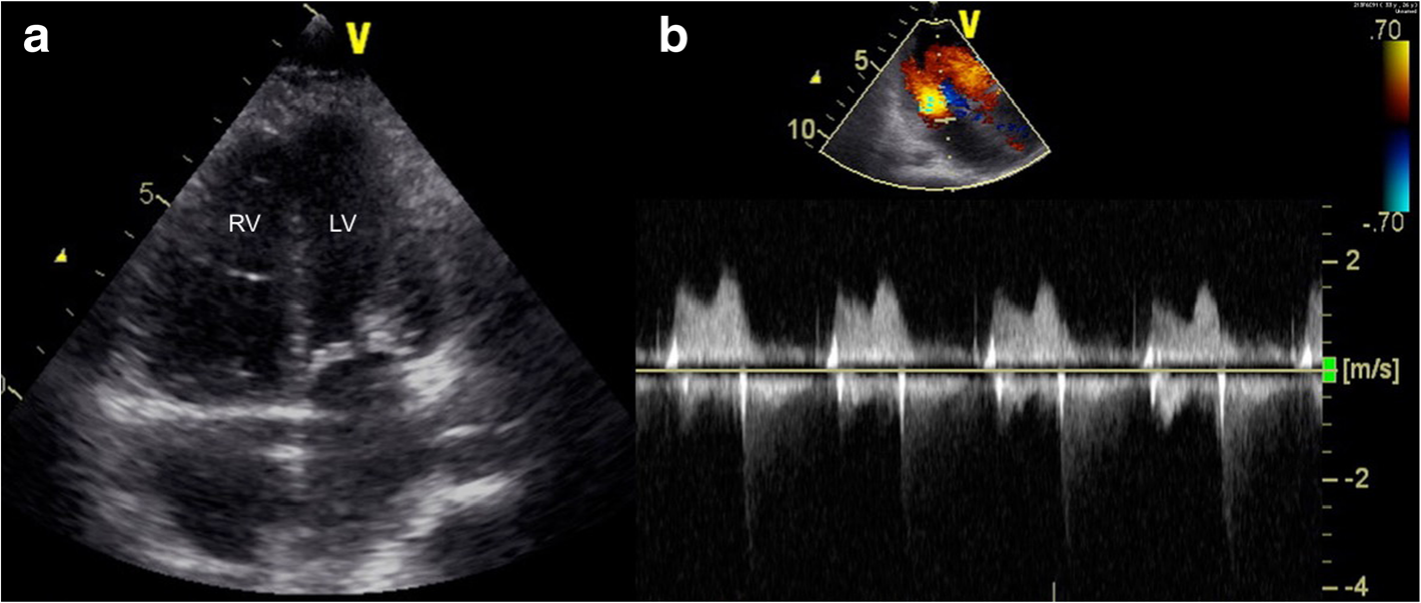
Echocardiogram in the apical view showing severe mitral stenosis with a mean pressure gradient of 10 mmHg with mild thickening of the mitral valve leaflets. a: B-mode image, b: Continuous-wave Doppler method. RV, right ventricle; LV, left ventricle
